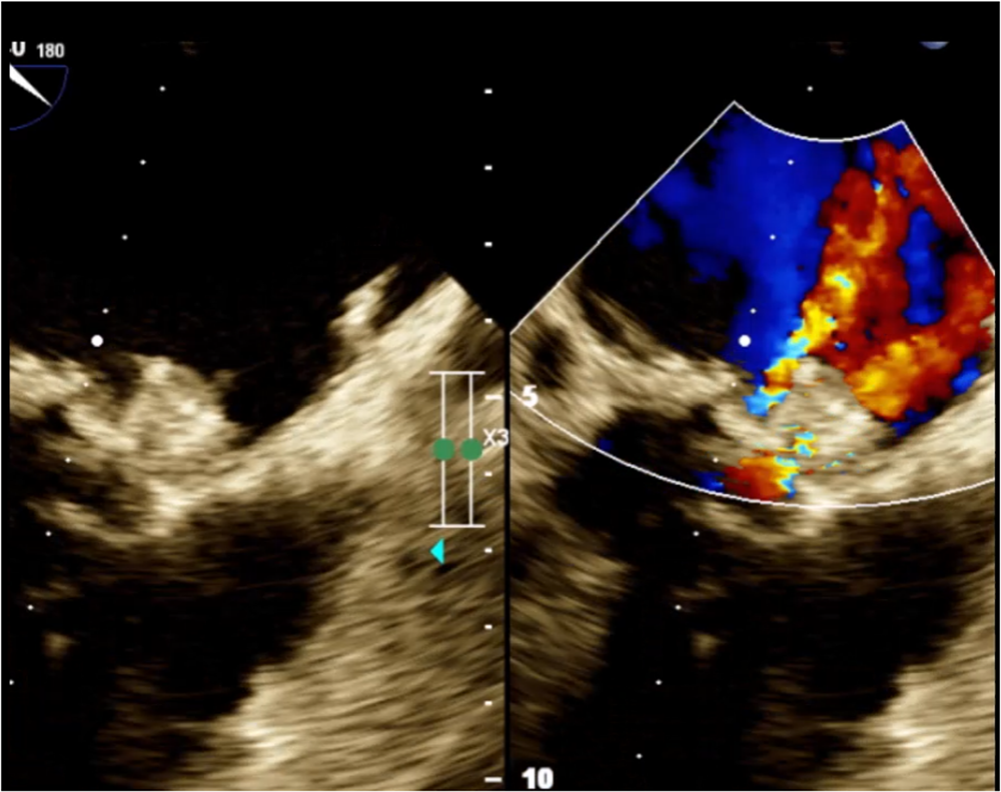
TEE showed mild residual paravalvular leakage. TEE, transesophageal echocardiography.
